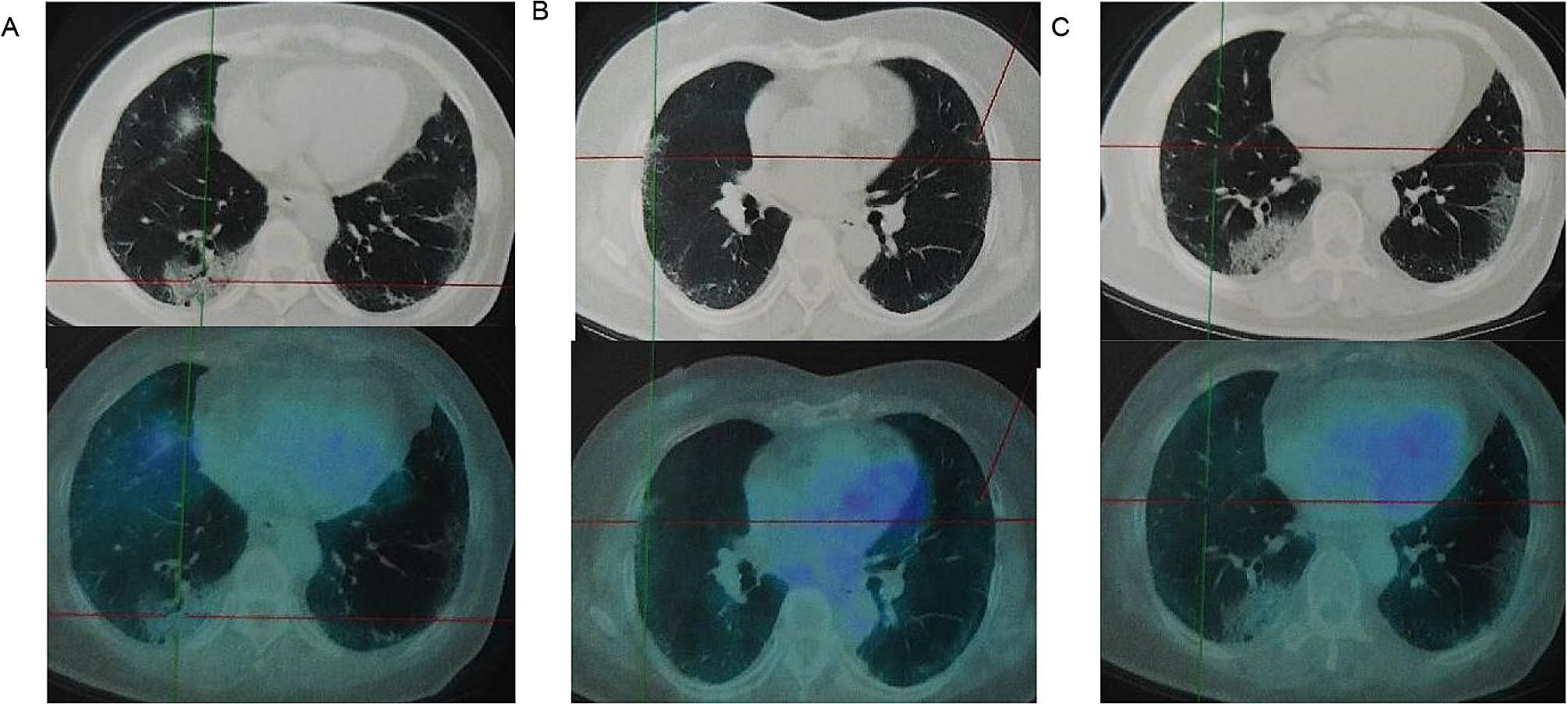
PET-CT revealed extensive interstitial changes and multiple patchy areas in both lungs with slightly increased fluorodeoxyglucose (FDG) metabolism (SUV = 1.95), and the larger lesions were in the inner and posterior basal segments of the right lower lobe
